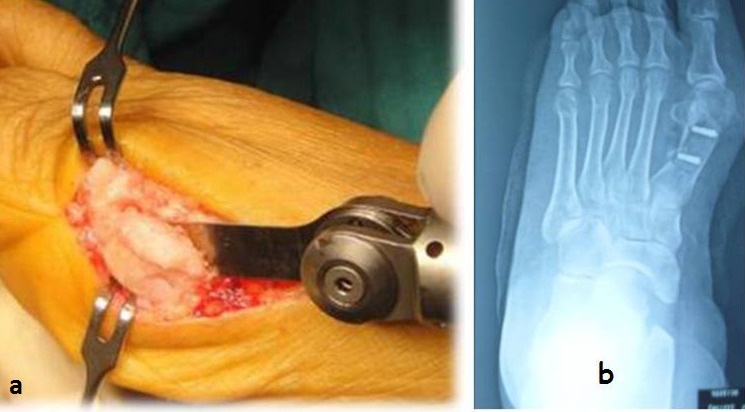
a) montrant voie d'abord chirurgicale ainsi que réalisation d'ostéotomie de Scarf; b) radiographie du pied montrant la fixation par deux vis de Scarf après ostéotomie de Scarf
